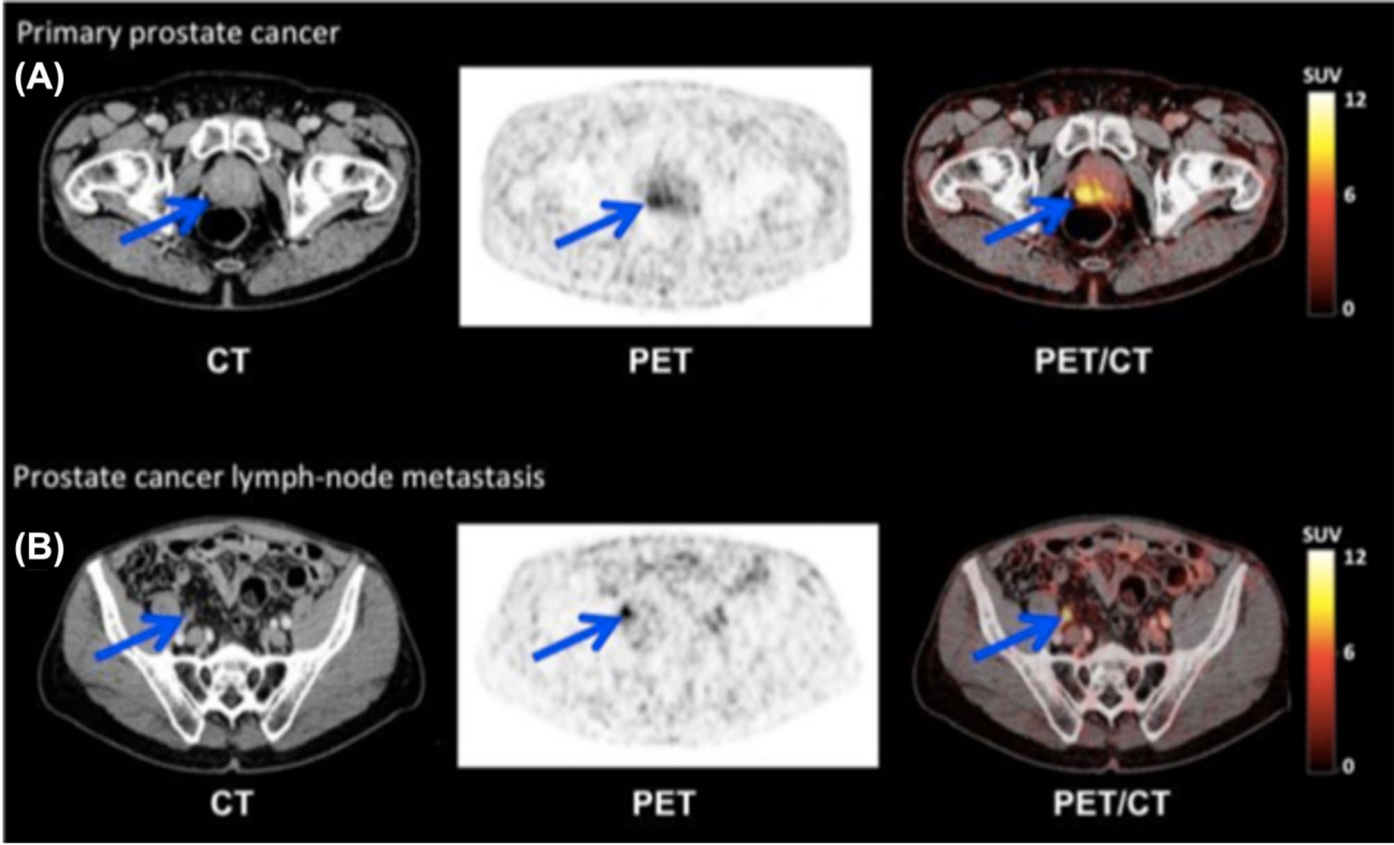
uPAR PET imaging in PCa. (A) Representative transverse CT, PET, and fused PET/CT images of a primary tumor lesion (blue arrows) showing high uptake of [^64^Cu]Cu-DOTA-AE105. (B) Images from another case depict a uPAR-positive regional lymph node metastasis (blue arrows) with similarly high tracer accumulation. Reproduced from Persson et al. [199], licensed under CC BY-NC-ND 3.0 (https://creativecommons.org/licenses/by-nc-nd/3.0/)
